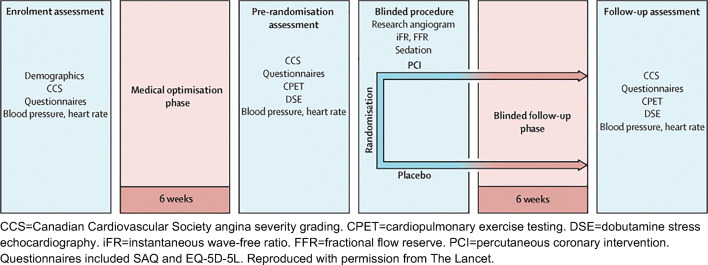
ORBITA study design. CCS = Canadian Cardiovascular Society angina severity grading, CPET = cardiopulmonary exercise testing, DSE = dobutamine stress echocardiography, iFR = instantaneous wave-free ratio, FFR = fractional flow reserve, PCI = percutaneous coronary intervention. Questionnaires included SAQ and EQ-5D-5L. Reproduced with permission from The Lancet
